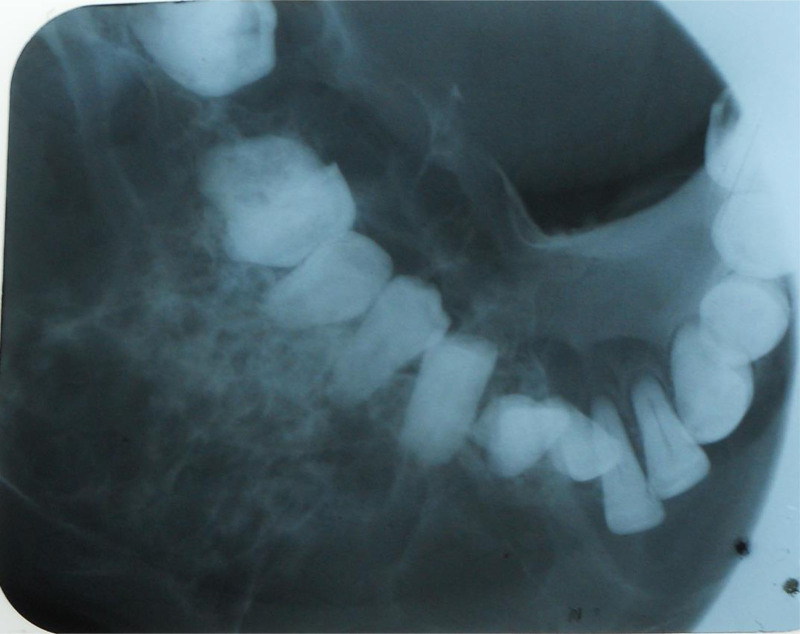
Occlusal radiograph showing a multilocular radiolucent lesion with cortical expansion and tooth displacement.
